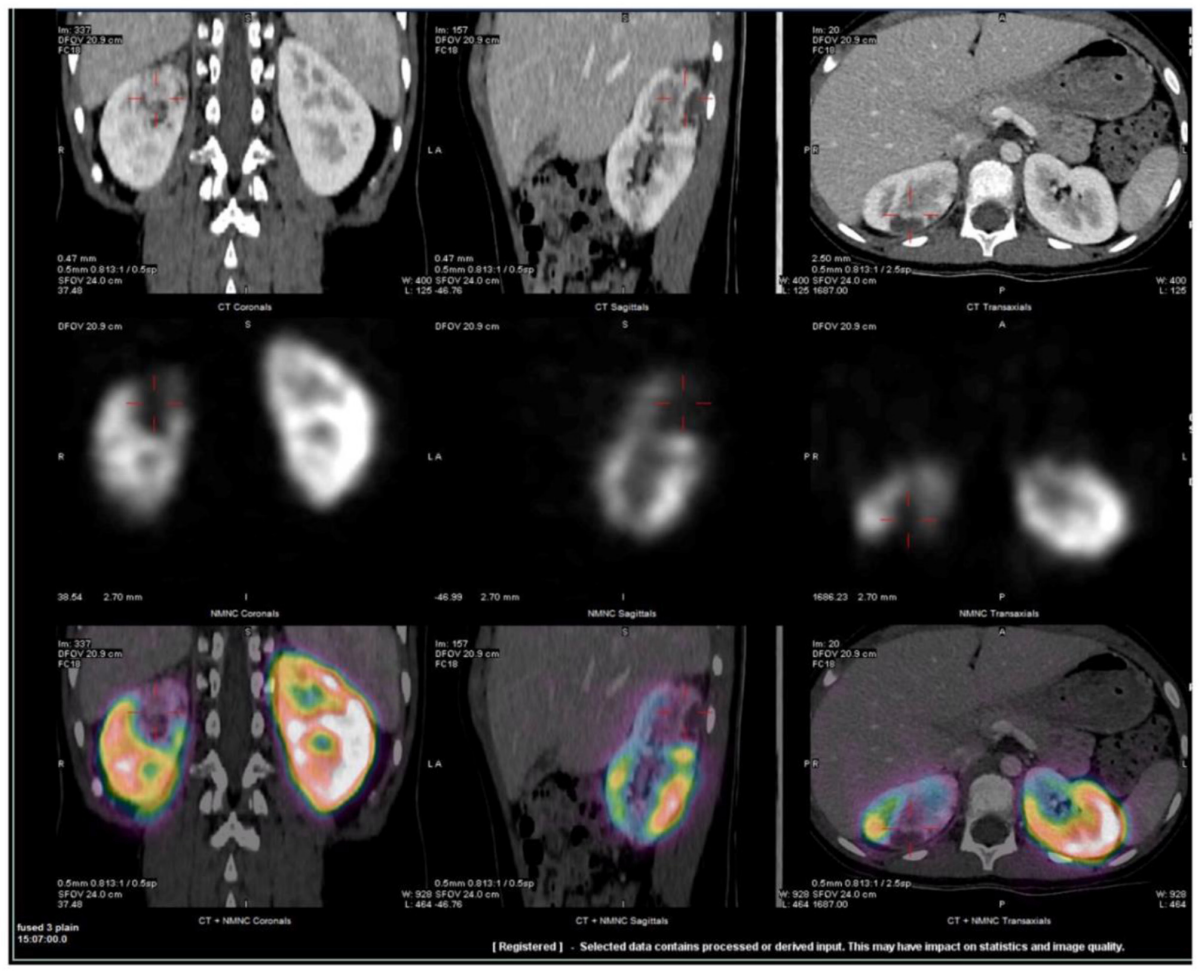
Representative fused SPECT/CT images of Tc99m-DMSA scan. CT scan was obtained with IV contrast. Images demonstrate wedge-shaped cortical defect involving the superior pole of the right kidney compatible with infarct. Two additional, smaller defects were also visualized in the lower and mid poles (not shown). Upper row: CT images, Middle row: Tc99m –DMSA scan and Lower row: fused SPECT/CT images in coronal, sagittal and axial projections.
